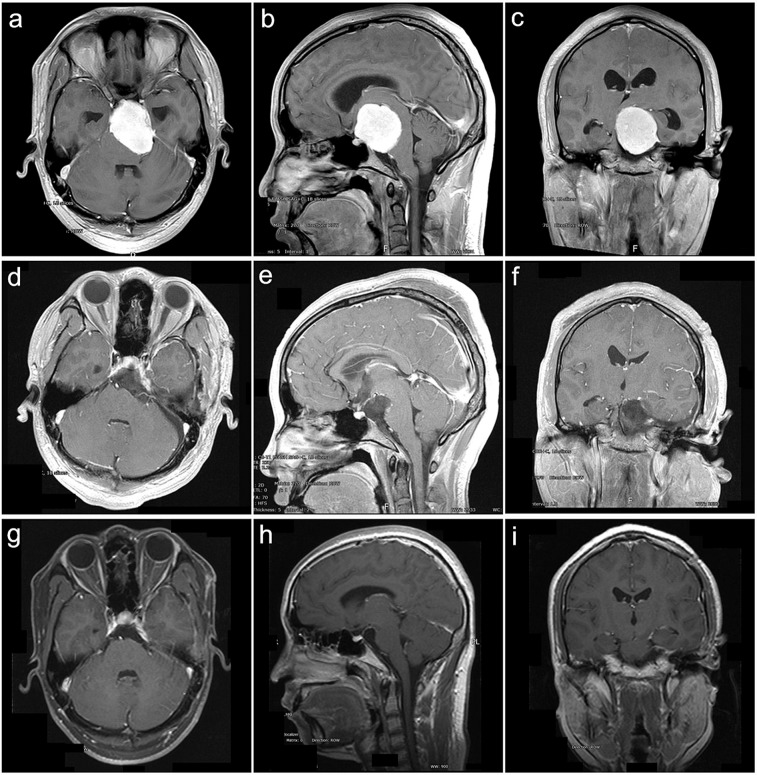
Petroclivosphenoidal type case 2. A 58-year-old female presented with headache and hobble for 8 months. The preoperative KPS score was 80. She was achieved GTR with the STTA of palsy in CN III, IV and V. The postoperative KPS score was 70. With a follow-up of 48 months, she participated in normal activities and had a KPS score of 90 without recurrence. (a–c) Preoperative MRI T1 contrast axial, sagittal, and coronal images. (d–f) Postoperative MRI T1 contrast axial, sagittal, and coronal images. (g–i) Follow-up MRI T1 contrast axial, sagittal, and coronal images.
